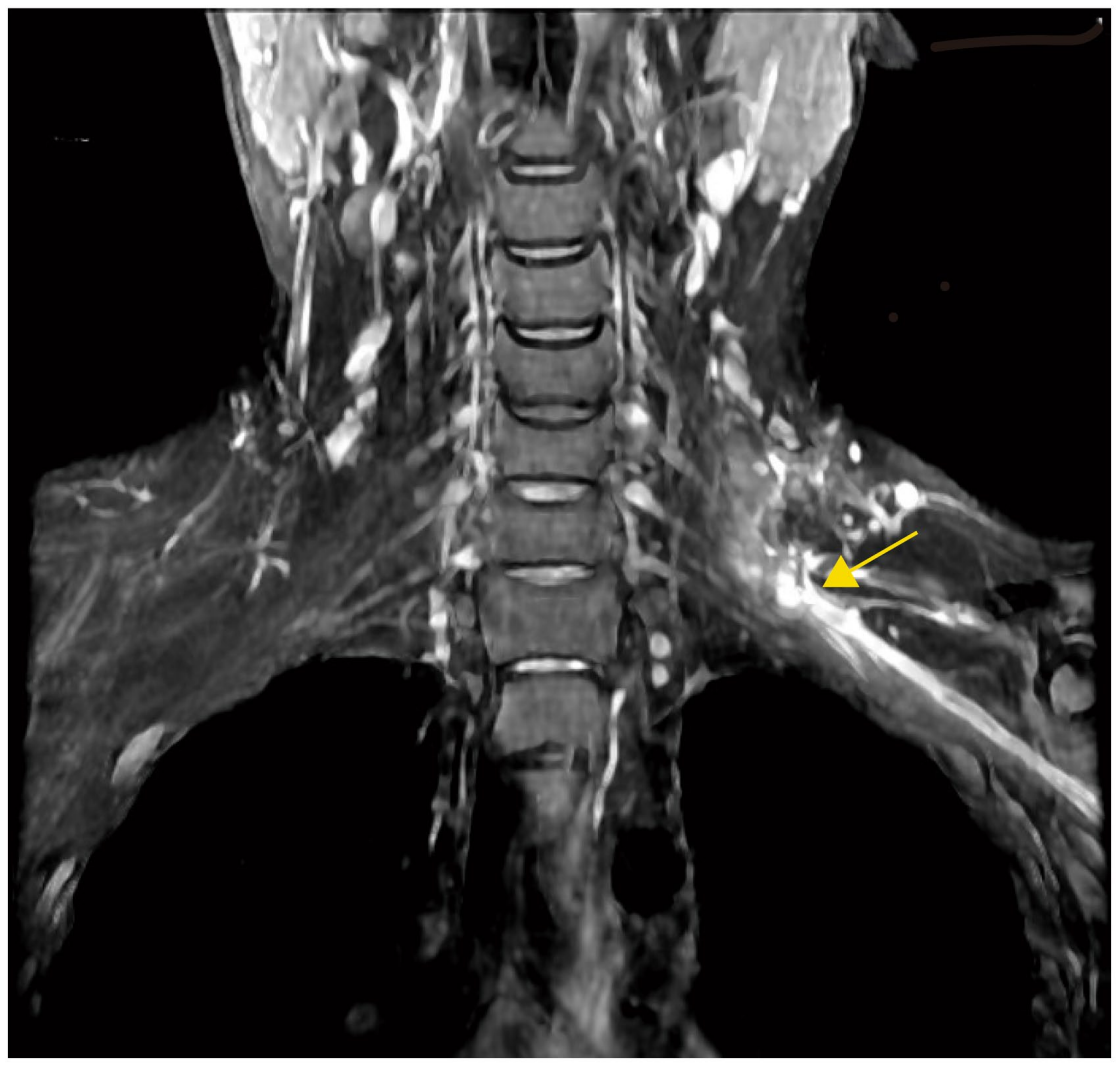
MRI image for the treatment of brachial plexus neuroma. The location of the neuroma after neuroanastomosis is marked with a yellow arrow.
